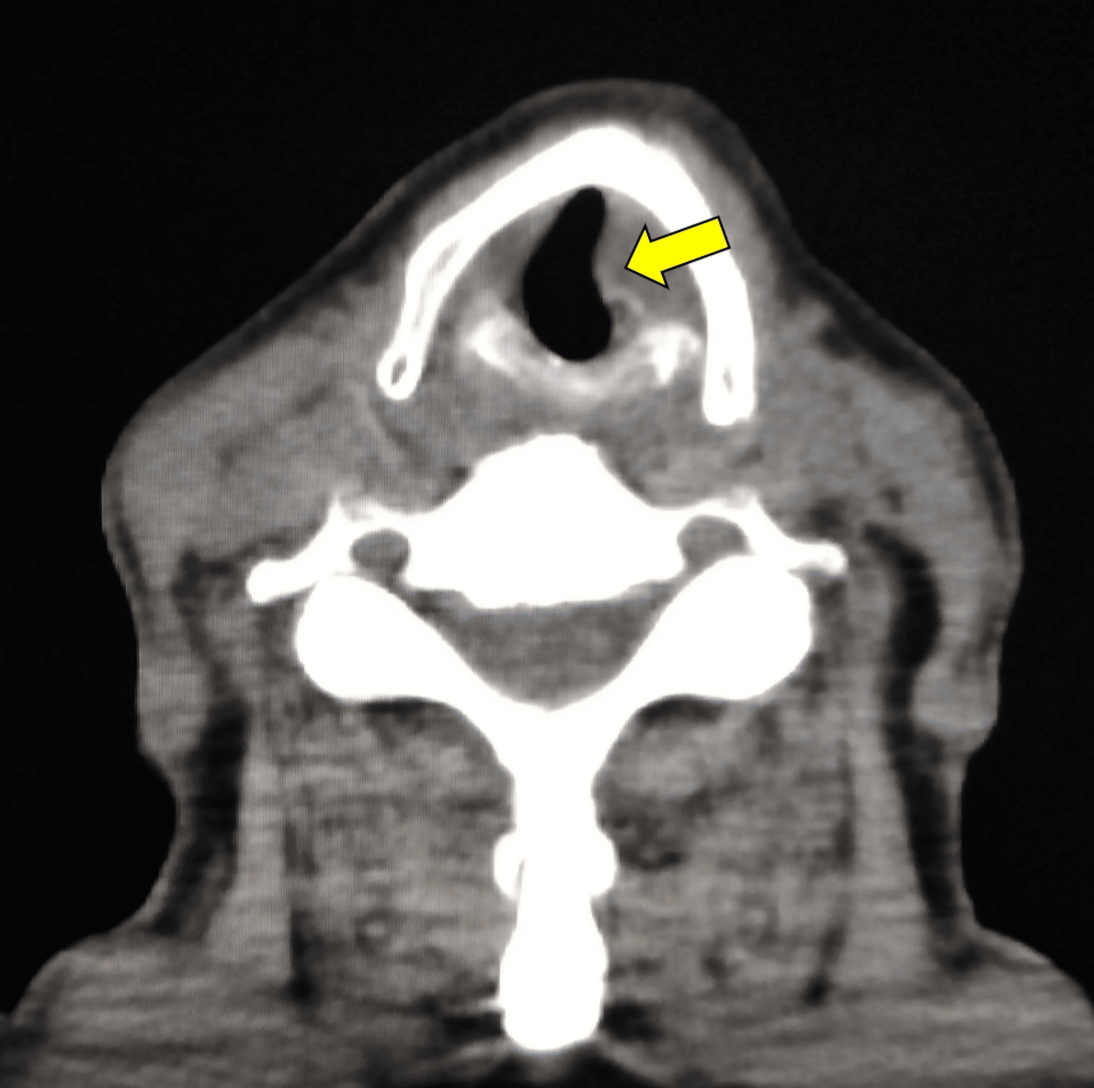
Computed tomography of the neck The picture of the slice on the vocal cord level showed an irregular shape of the edge in the left vocal cord compared to the right one (yellow arrow).
